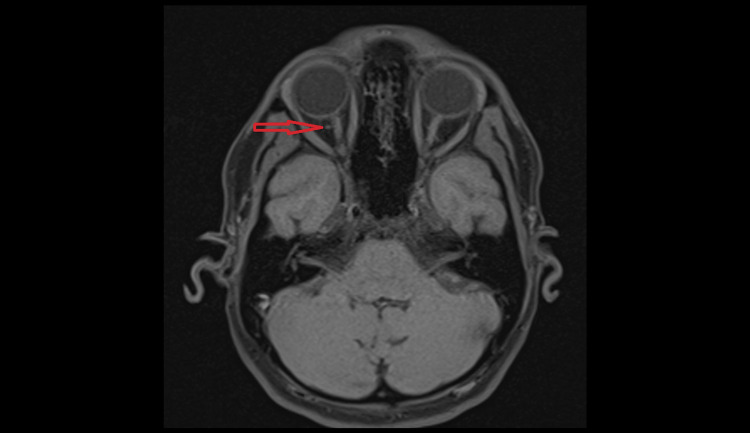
Axial view of a T1-weighted MRI pre-contrast showing an abnormal round structure (red arrow) located just behind the right globe and lateral to the optic nerve
